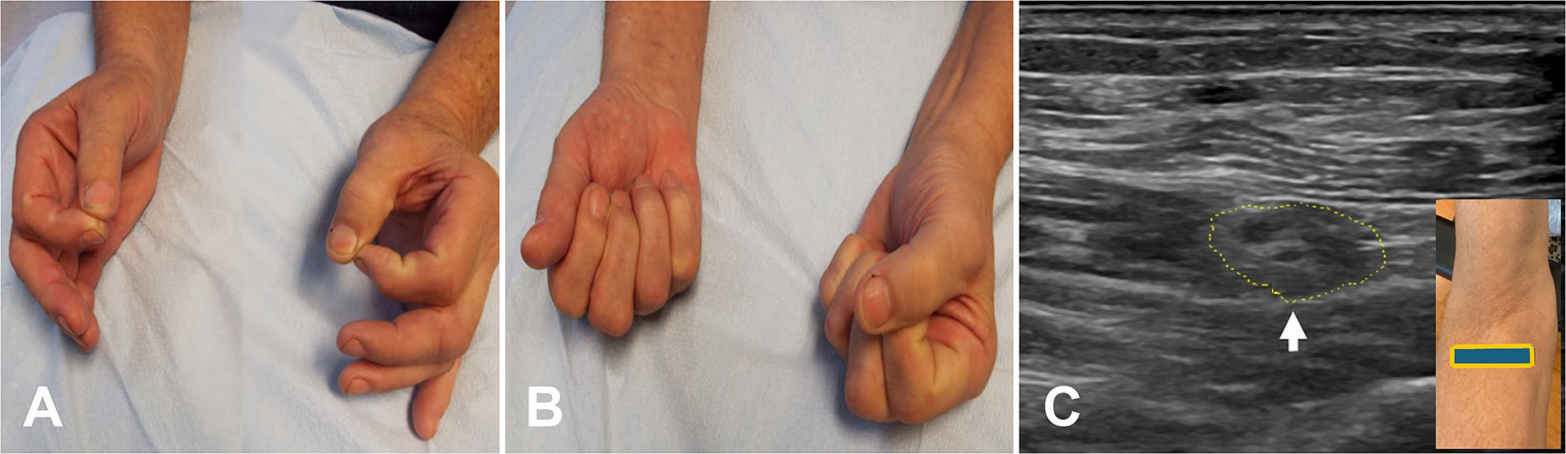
Acute onset of pronator teres syndrome. (A) Positive O sign on the right. (B) Weakness of the flexor pollicis longus on the right. (C) Ultrasound study showing enlarged median nerve (encircled by yellow dots, arrow) with a few large fascicles.
